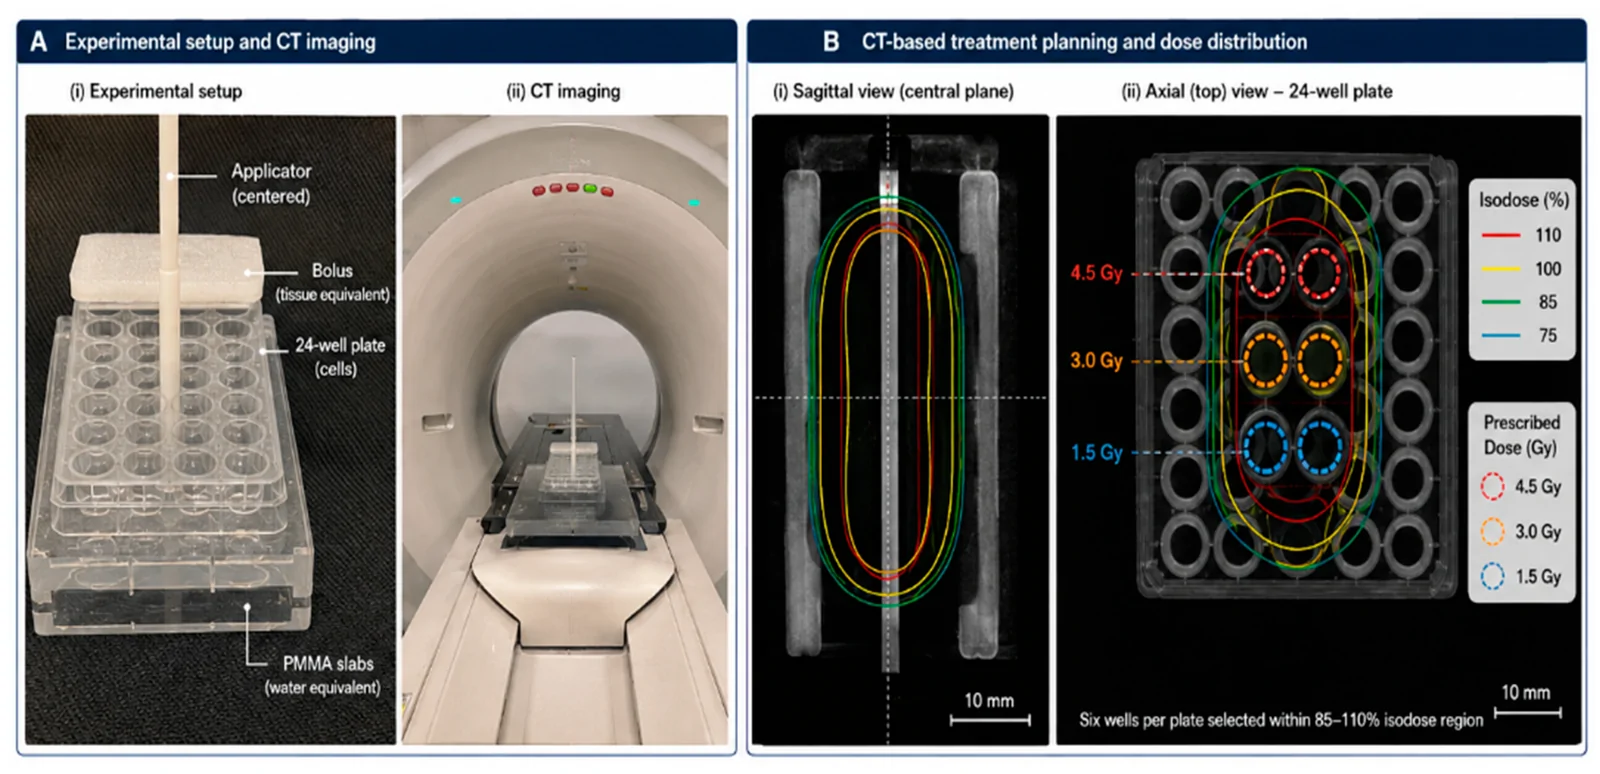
Experimental HDR brachytherapy setup and CT-based dose distribution. (A) Experimental irradiation configuration showing the 24-well culture plate positioned between PMMA tissue-equivalent slabs, with a centrally placed applicator and overlying bolus, together with CT imaging of the setup for treatment planning. (B) CT-based reconstruction and dosimetric planning performed in the Oncentra Brachy treatment planning system. Isodose distributions are shown in sagittal and axial views. Six wells per plate (dashed circles) were selected within the 85–110% isodose region to receive the prescribed doses of 1.5, 3.0, and 4.5 Gy delivered using an HDR ^192^Ir source.
